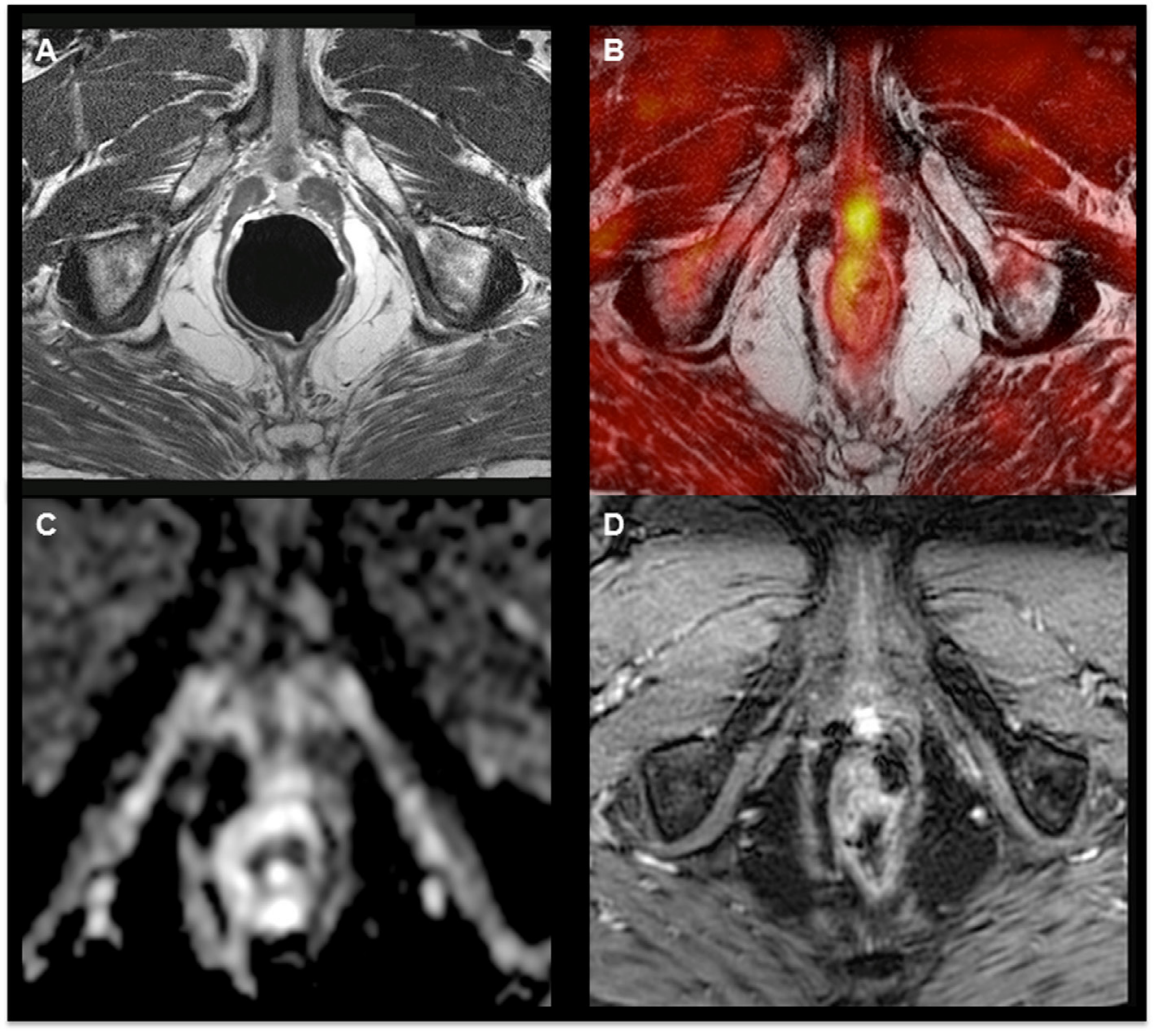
^18^F-Fluorocholine hybrid PET–MRI images showing hyperintensity on the T2-weighted sequence (A) and focal hypermetabolism (B) in a nodule with limited diffusion restriction on ADC map (C) and hyperperfusion (D) in a patient with a biochemical relapse (PSA = 1.75 ng/mL, doubling time = 11 months) 9 years after radical prostatectomy.
